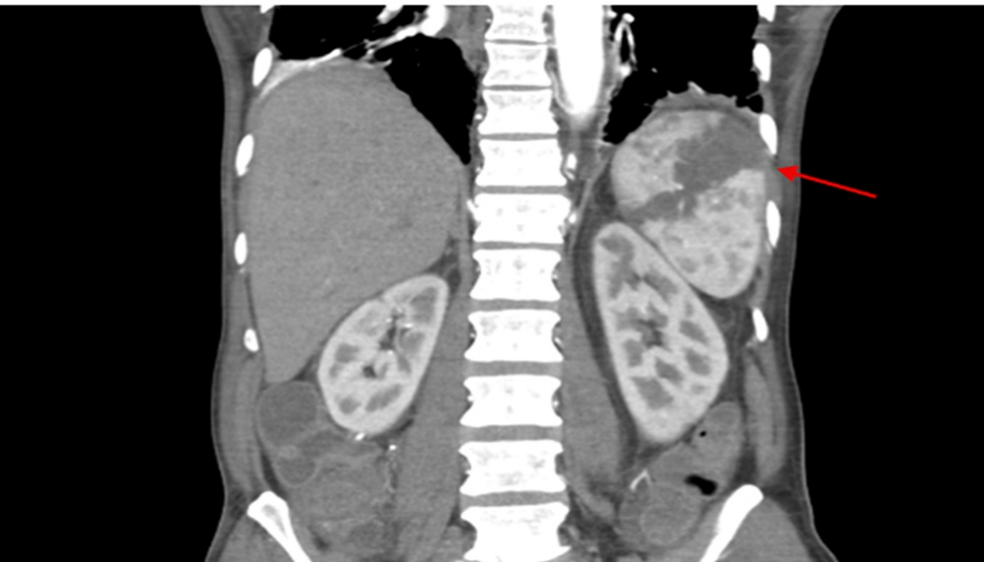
Coronal plane contrast CT of the abdomen and pelvis, with a red arrow highlighting splenic infarction.
